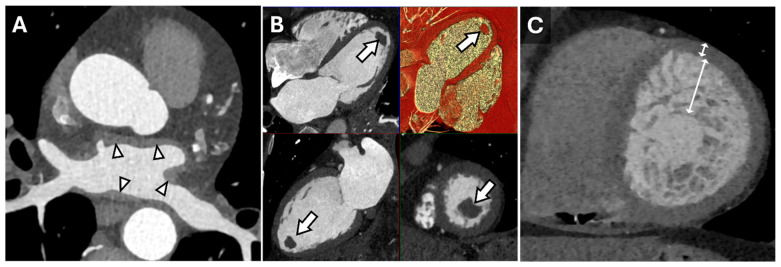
Cardiac Findings and CT Imaging. Axial reconstruction from a contrast-enhanced cardiac CT (A) demonstrating irregular left atrial wall thickening (arrowheads) reflecting amyloid deposition in the setting of cardiac amyloidosis. Multiplanar (HLA, VLA, SAX, 3D) diastolic images (B) from a contrast-enhanced cardiac CT in a patient with a left ventricular apical thrombus (arrows). Short-axis end-diastolic image from a contrast-enhanced cardiac CT in a patient with myocardial non-compaction (C) demonstrating very prominent trabeculation of the mid-apical left ventricle with an increased ratio of non-compacted to compacted myocardium measuring >> 2.3:1 (double-headed arrows).
